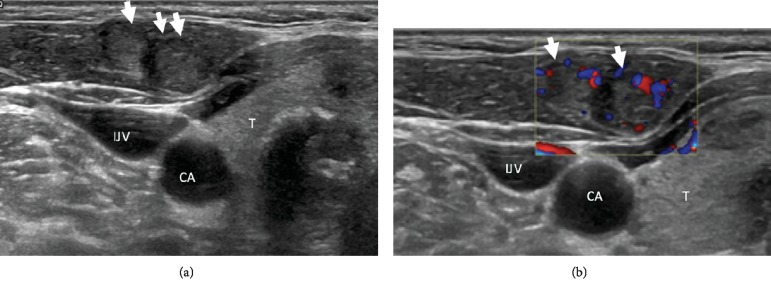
Arrows point the 2 nodules within the right sternocleidomastoid muscle on ultrasound. IJV, internal jugular vein; CA, carotid artery; T, thyroid.
